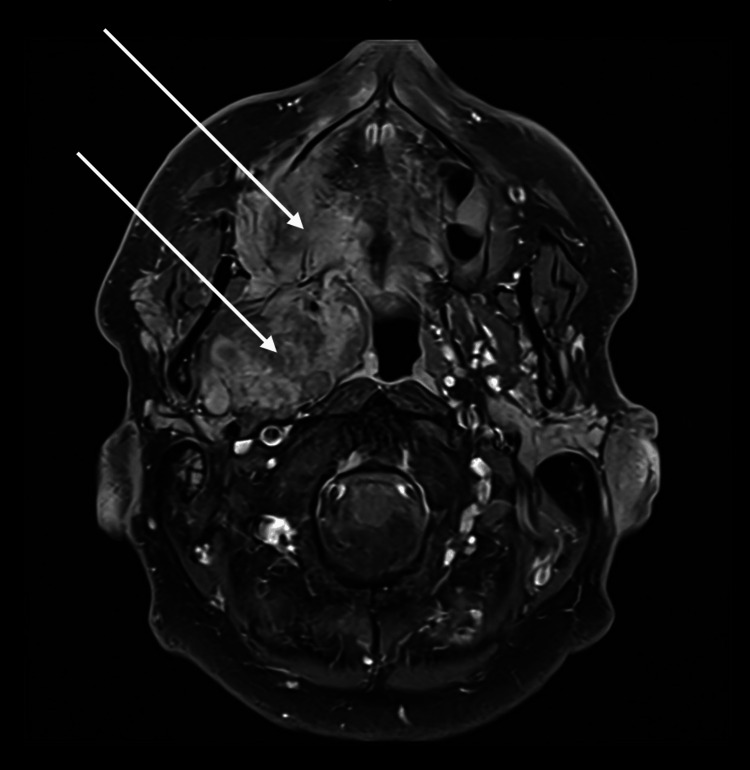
MRI demonstrating a large, locally invasive aggressive maxillary tumour involving the nasopharynx. There is bony destruction with soft tissue extension into the masticator space, pterygopalatine fossa, nasal cavity, and inferior orbital wall (arrows).
